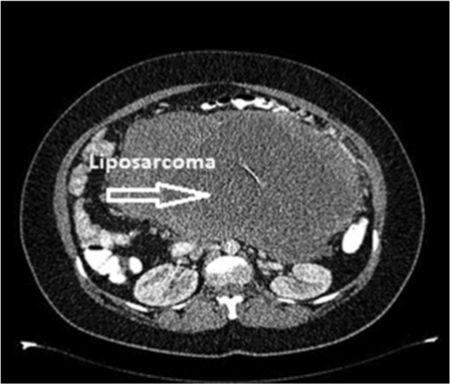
CT image of grade 3 liposarcoma in size of 30 cm (pleomorphic type).
